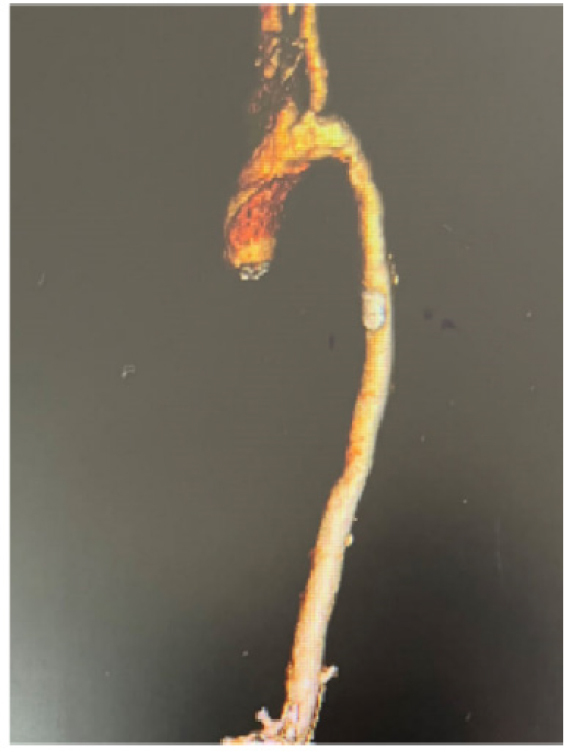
Aortic CTA of the case 2. CTA, computed tomography angiography.
